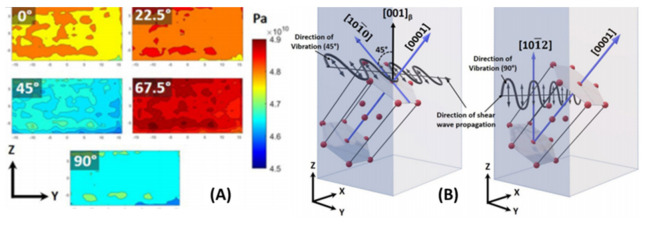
(A) Ultrasound scanned angle-dependent dynamic Young’s modulus distribution. (B) Hypothesis based on the experimental observation from ultrasonic techniques and conventional characterizations.
